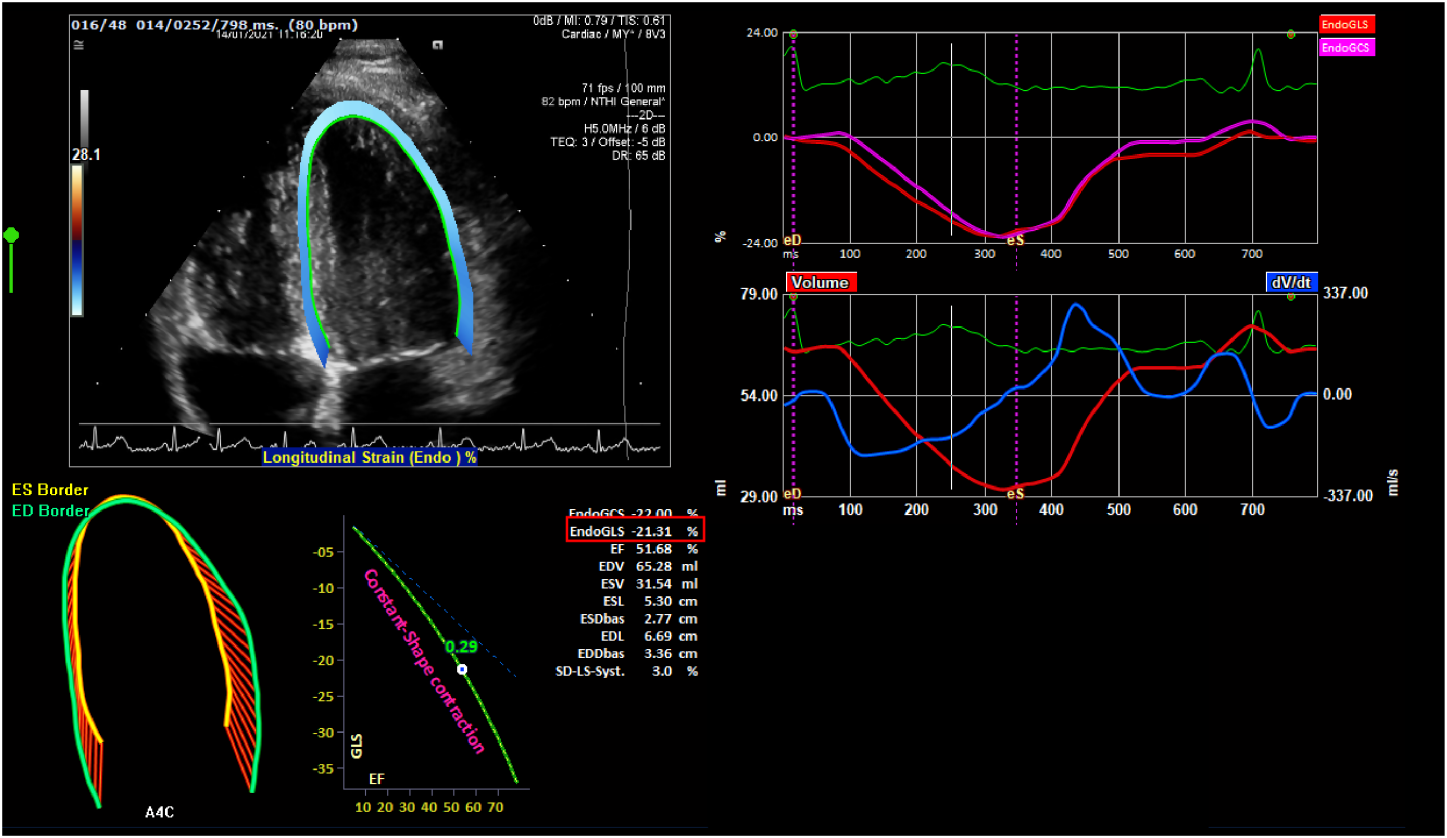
A depiction of strain echocardiography. The endocardial global longitudinal strain (endo GLS), represented as a percentage derived from the average of 6 segments of interest within the left ventricle, is highlighted in red.
